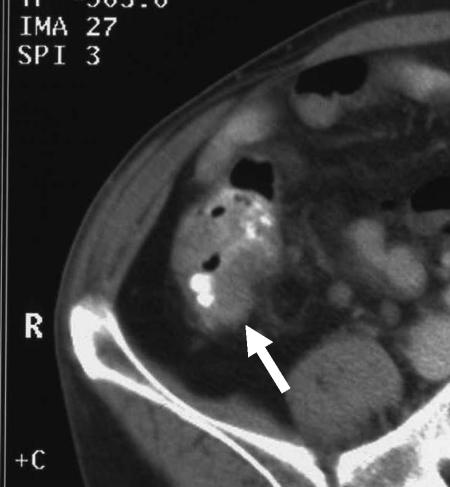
Preoperative CT scan showing a fungating tumour of the ascending colon. The colonic wall is thickened and the posterior contour is irregular owing to tumour projection beyond the non-peritonealised muscle coat. As there is no tumour involvement of the peritonealised surfaces, this is considered a relatively good prognosis T3 tumour. Pathology confirmed a pT3 tumour of the ascending colon.
